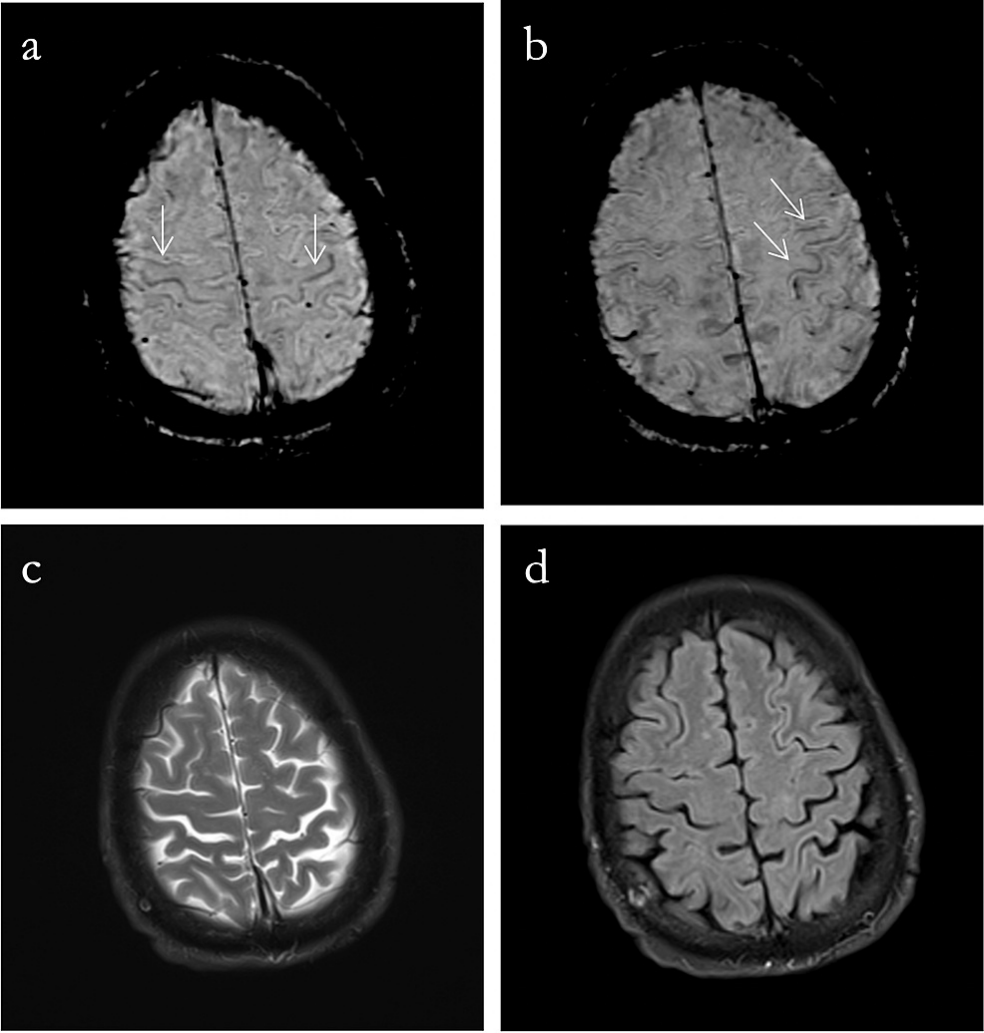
Brain MRI Axial susceptibility-weighted imaging images (a, b) show the classic “motor band sign,” which are curvilinear bands of low signal intensity solely observed in the primary motor cortex bilaterally (white arrows). Note that this finding is not seen on the corresponding axial T2 image (c). Axial fluid-attenuated inversion recovery image (d) shows subtle signal changes in the subcortical white matter, more on the left side, indicating the degeneration of the corticospinal tract MRI: magnetic resonance imaging
